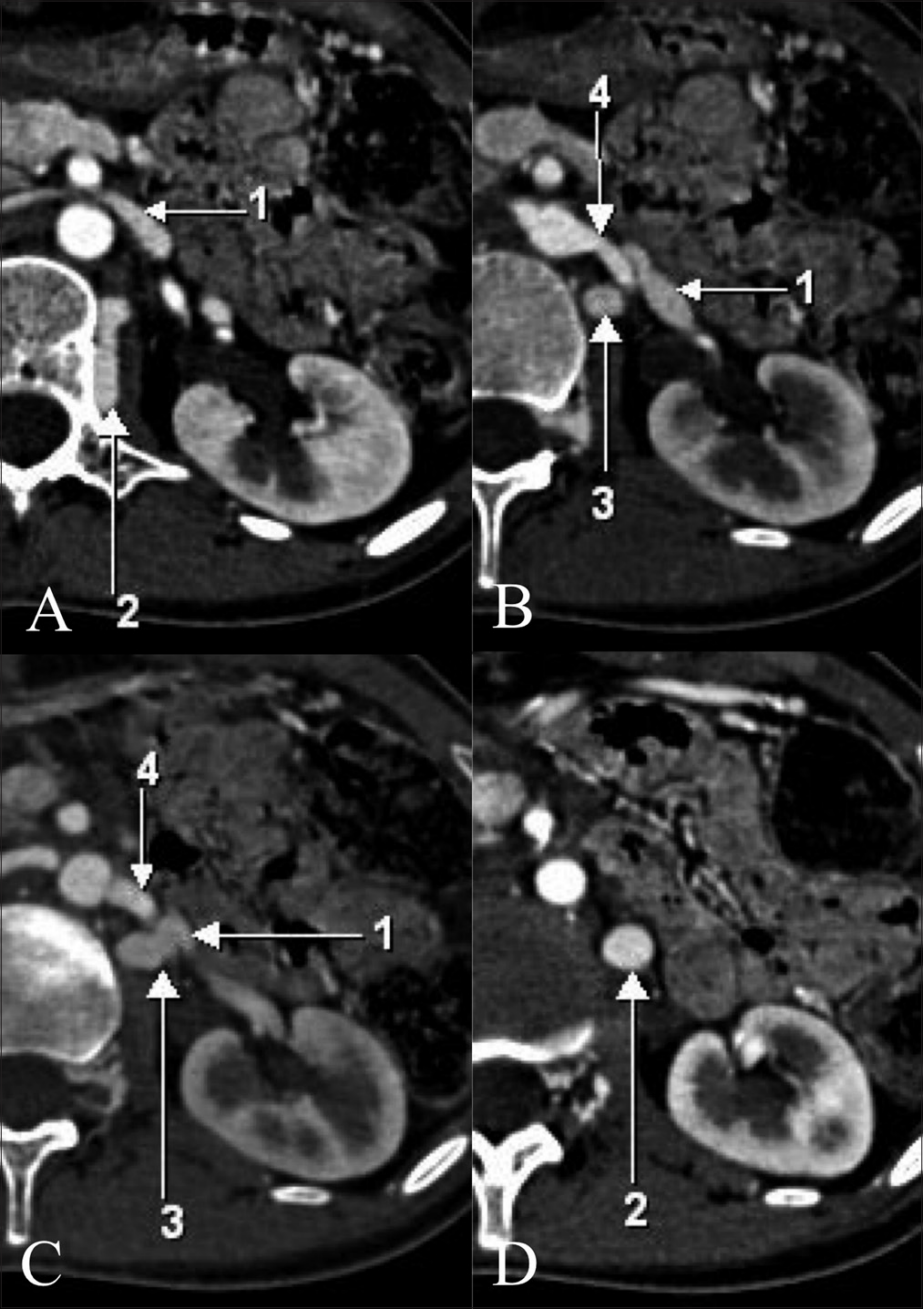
Narrow collimation, sequential, axial contrast-enhanced CT scans showing the relationships of the various vessels. Arrow 1 - left renal vein, arrow 2 - communicating vein between the left renal vein and the left ascending renal vein, arrow 3 - left ascending lumbar vein, arrow 4 - left renal artery
